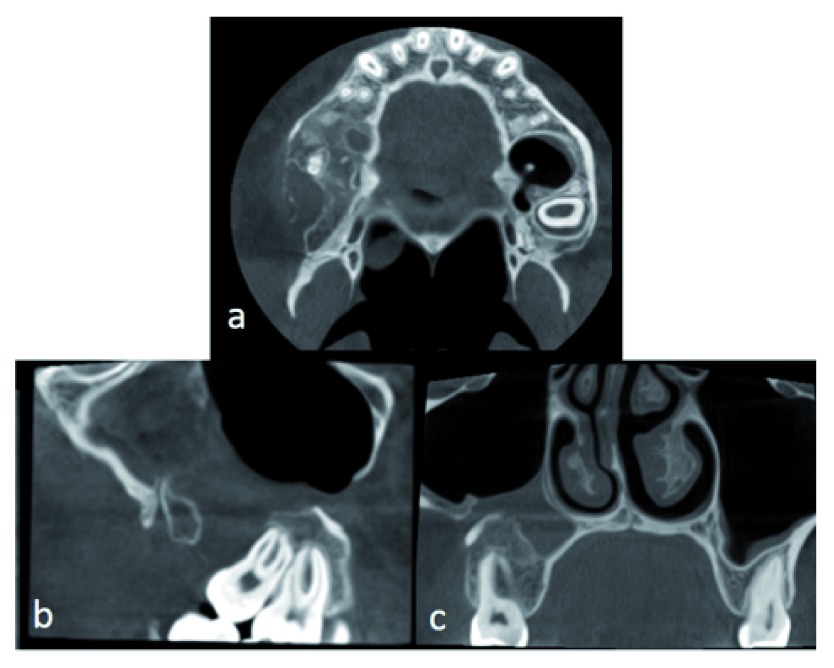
Multiplanar cone beam computed tomography (CBCT) slices: (
